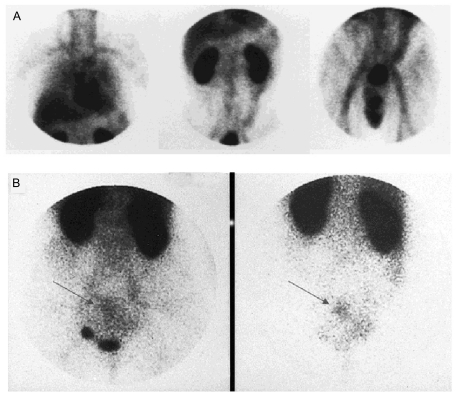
SPECT imaging with CEA-Scan. A) Normal biodistribution of CEA-Scan at 4 h post-injection. Anterior planar images of the chest, abdomen and pelvis are shown. B) Planar anterior pelvis imaging at 5 h (left) and 24 h (right) post-injection clearly delineated the lesion. Arrows point to the tumor which was close to the bladder. Adapted from (Erb and Nabi, 2000).
